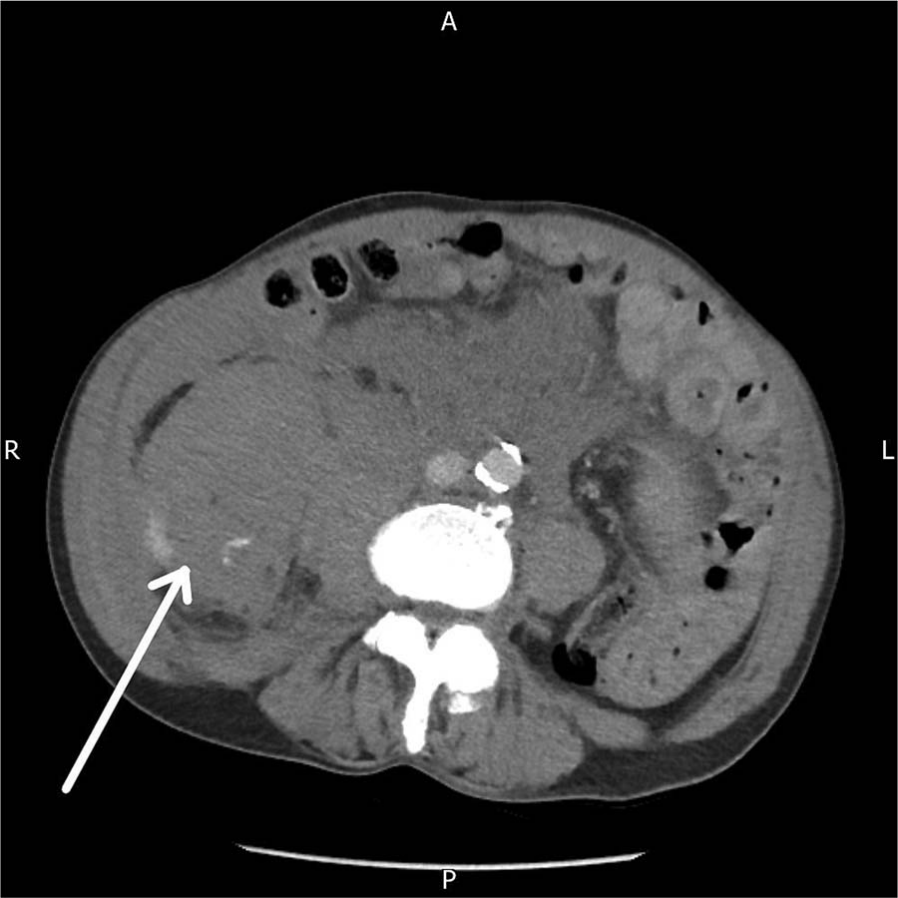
Axial contrast-enhanced CT abdomen and pelvis demonstrating a right-sided retroperitoneal haematoma with evidence of active contrast extravasation.
